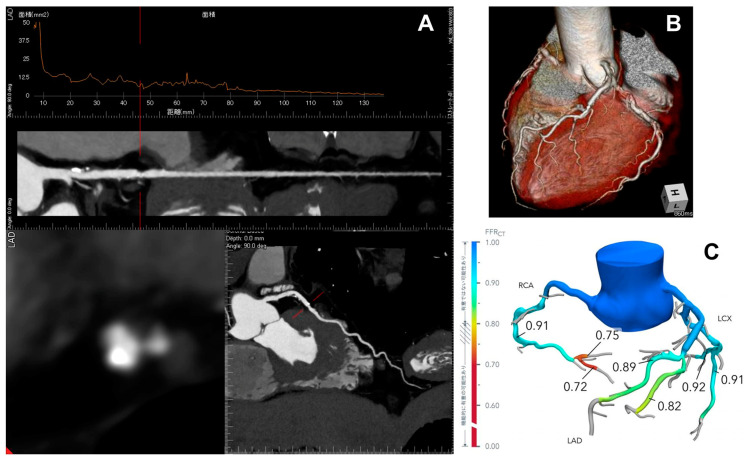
Quantitative computed tomographic coronary angiography (CTCA) (A), three-dimensional CTCA image (B), and fractional flow reserve (FFR-CT) measurement (C) in a 78-year-old male patient before surgery for a thoracic aortic aneurysm show moderate coronary lesions (50–70% lumen narrowing by visual CTCA) with partly calcified plaques in the right coronary artery (RCA) and left anterior descending artery (LAD). The RCA showed an FFR-CT value of 0.72 and was therefore grafted, whereas the LAD showed an FFR-CT value of 0.89 and was deferred.
